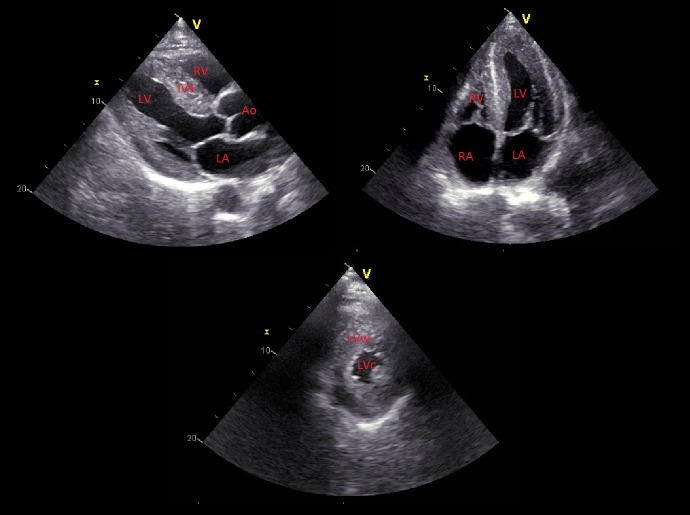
Echocardiogram Echocardiogram showing left ventricular hypertrophy and myocardial sparkling with an interventricular septum of 16 mm, a small left ventricular cavity with bi-atrial enlargement.
